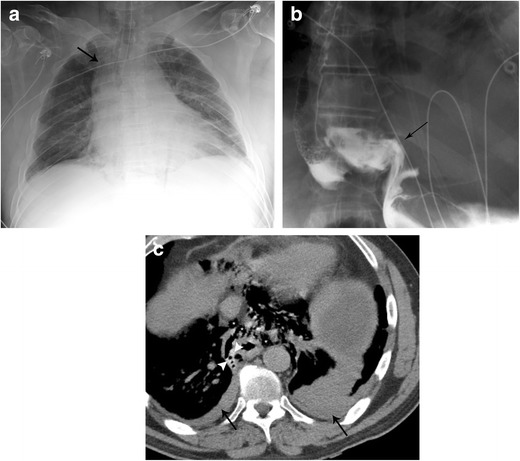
Boerhaave Syndrome. a AP chest radiograph shows pneumomediastinum (arrow). b Esophagram in AP projection demonstrates contrast extravasating from the distal oesophagus. c CT of the chest with oral contrast in soft tissue window demonstrates extraluminal contrast (white arrowheads), pneumomediastinum (*), and bilateral pleural effusions (arrows) in a patient with Boerhaave Syndrome
